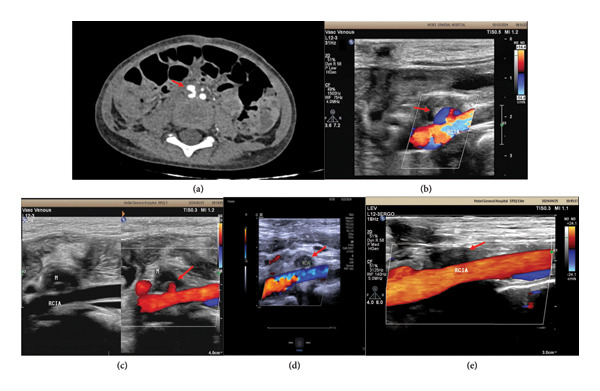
(a) February 9, 2024. Abdominal enhanced CT revealed rupture of the anterior wall of the right common iliac artery with pseudoaneurysm formation. (b) February 13, 2024 (Day 4), color Doppler ultrasound of the iliac artery demonstrated stable pseudoaneurysm dimensions, partial intraluminal thrombosis, and reduced flow cavity volume. (c) February 27, 2024 (Day 18), follow‐up iliac artery color Doppler ultrasound demonstrated significant reduction in the pseudoaneurysm cavity size. (d) March 22, 2024 (Day 42), follow‐up iliac artery color Doppler ultrasound demonstrated complete thrombosis of the pseudoaneurysm. (e) April 25, 2024 (Day 72), follow‐up imaging revealed complete resolution of the iliac artery pseudoaneurysm.
